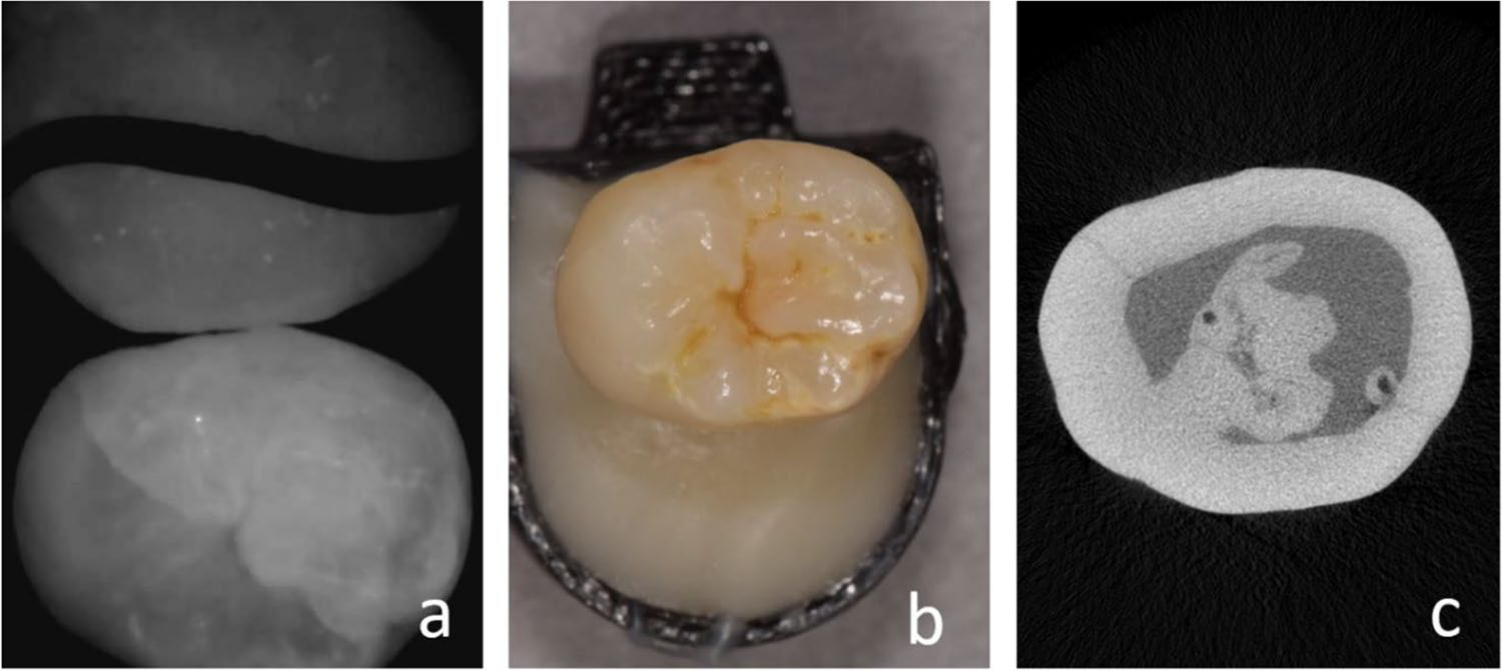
The diagonal crack extending through the clinical crown of a premolar cannot be identified with white light (*b*) but can be detected by near-infrared reflection (*a*) and micro-computed tomography (*c*)
